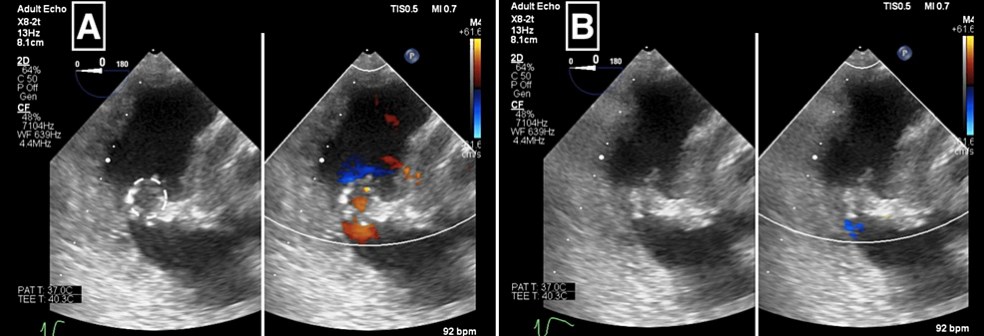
Transgastric TV short-axis view from intraoperative TEE after cone reconstruction of the TV. The images show diastole (A) and systole (B). The dotted line indicates the area corresponding to the TV orifice. TEE: transesophageal echocardiography; TV: tricuspid valve
